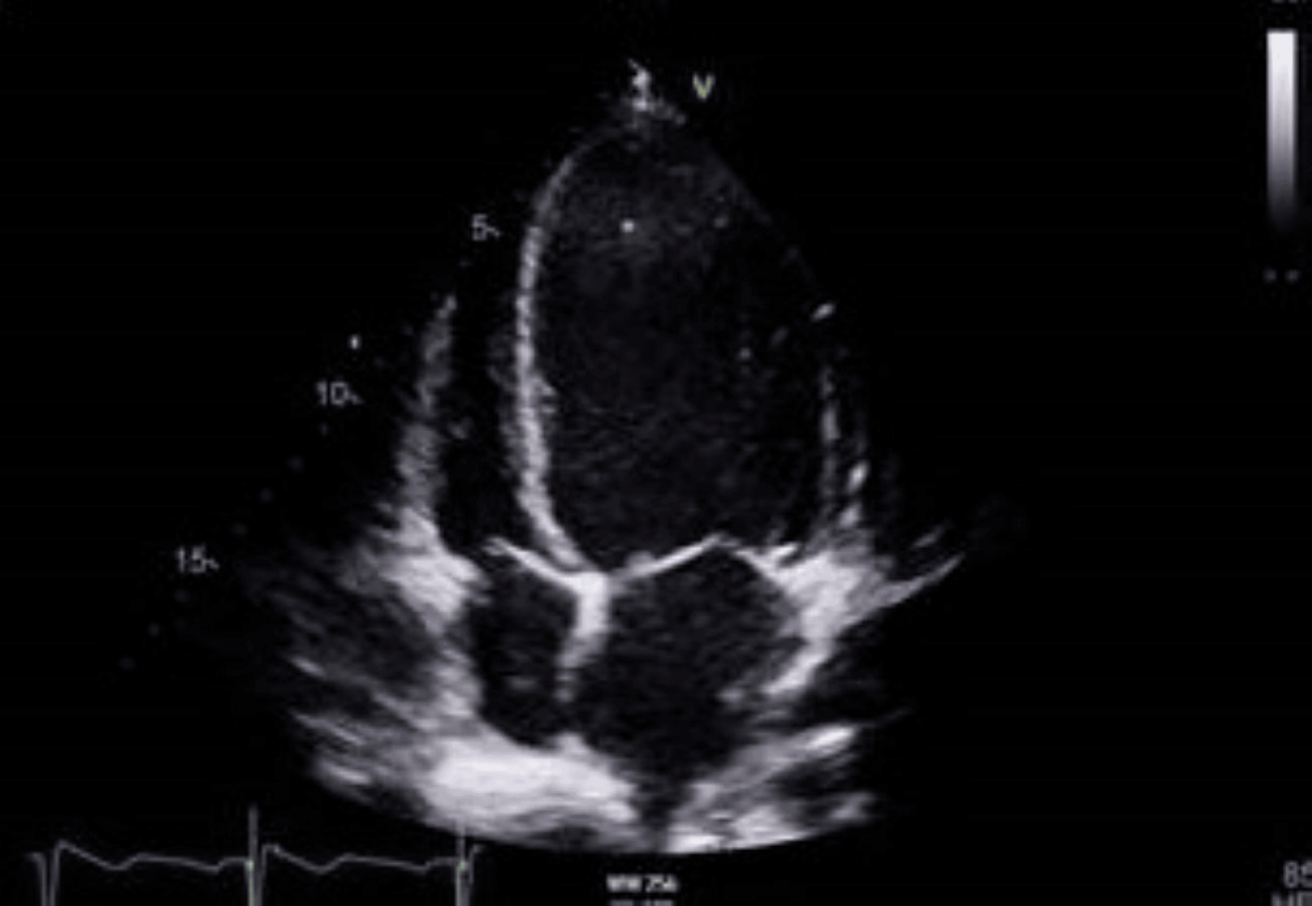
Transthoracic echocardiogram showing slightly dilated right atrium
